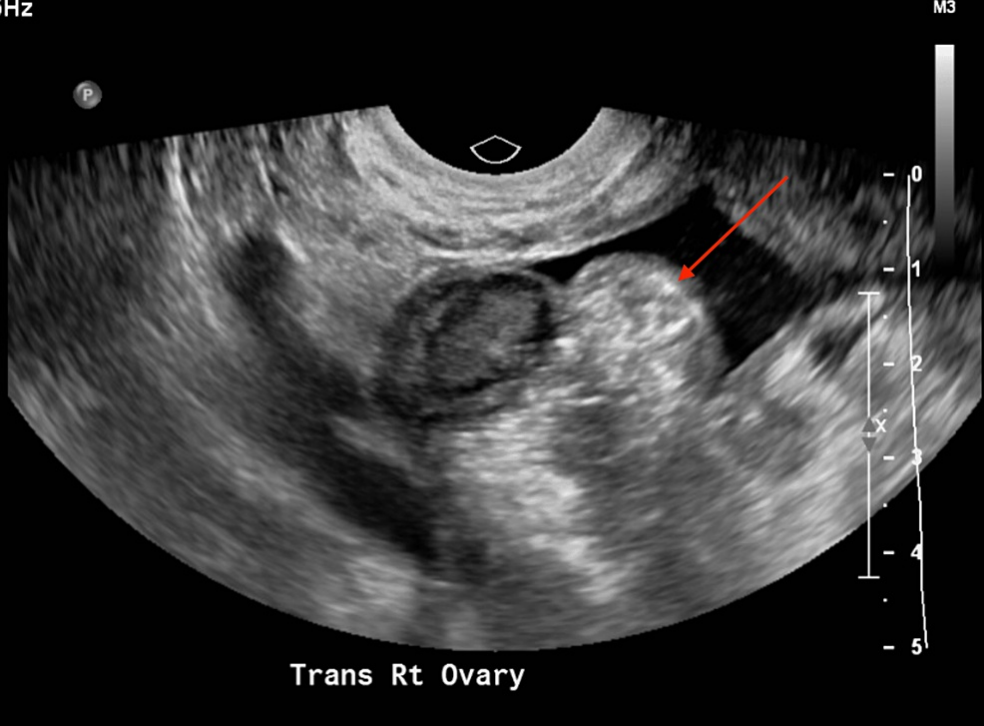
Transvaginal pelvic ultrasound showing right ovary with adjacent heterogeneous soft tissue structure.
